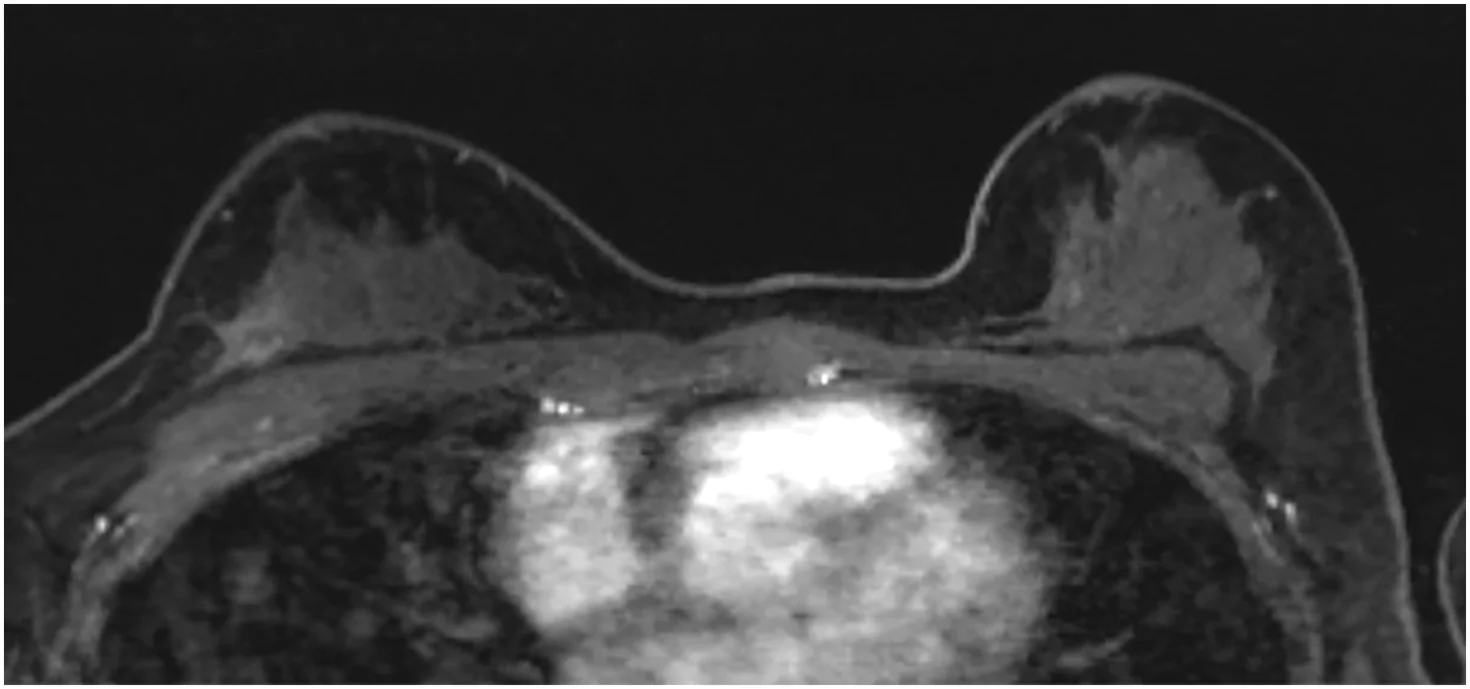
Contrast-enhanced MRI findings (after completion of neoadjuvant chemotherapy). Marked tumor shrinkage was noted, and no enhancement was observed.
